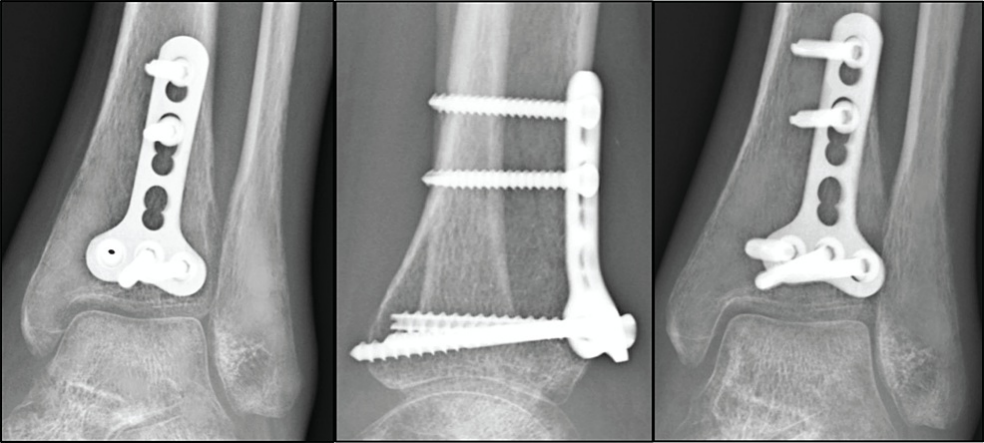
X-rays done at 12 weeks showing complete union at the fracture site
